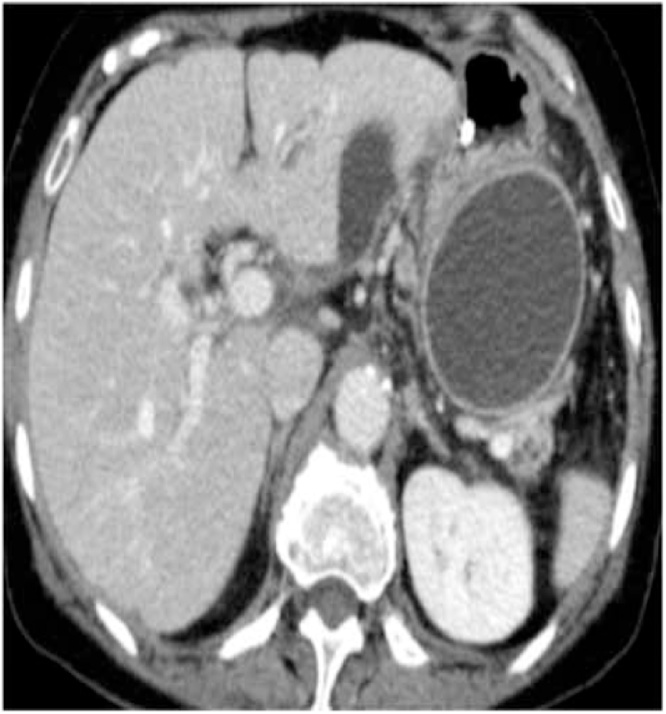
CT scan showing cyst In left hypochondrium (case 3).
